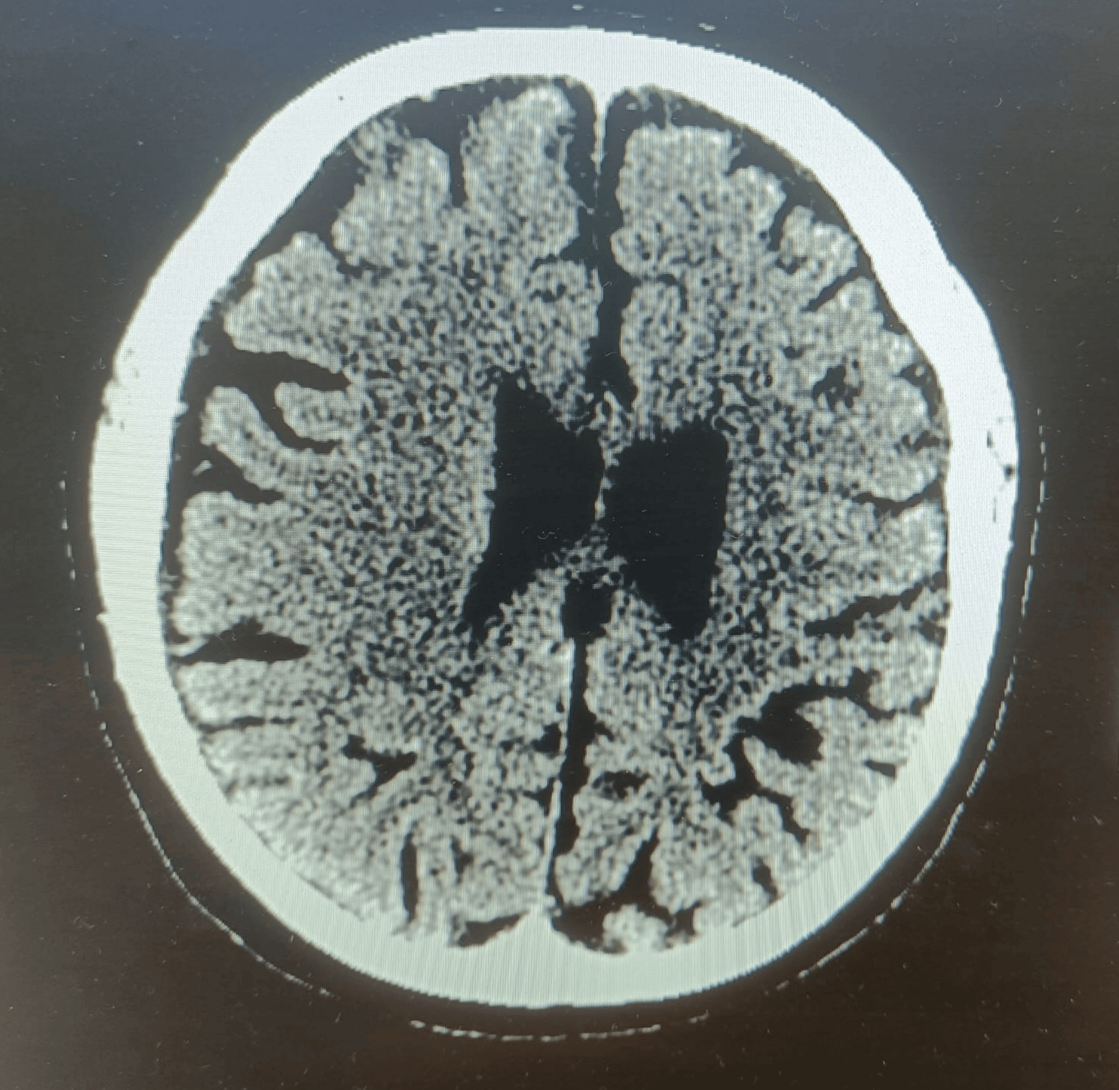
Non-contrast CT of the head demonstrates no acute intracranial abnormality.
